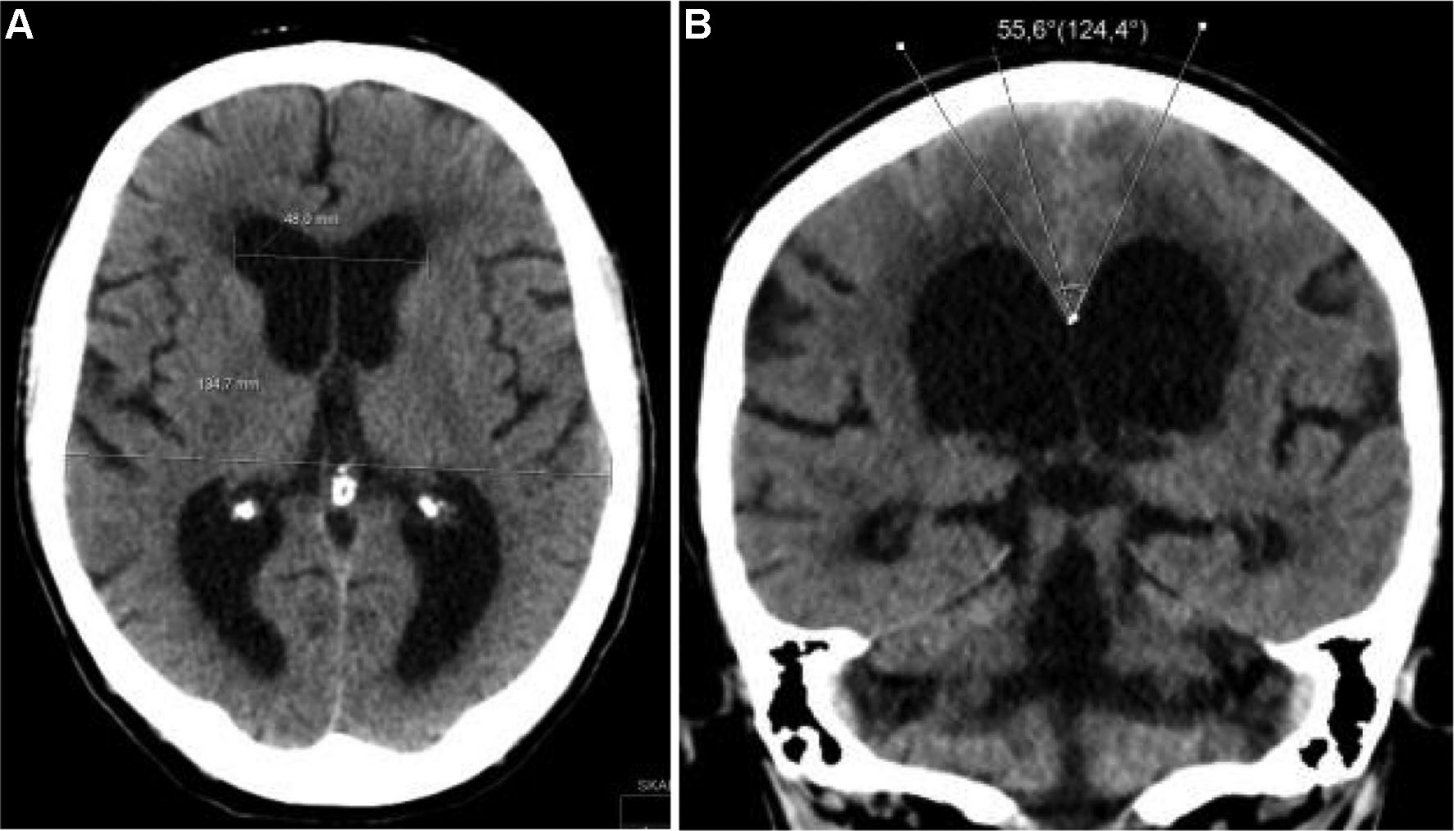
Radiological markers of NPH shown on CT scan a Evans’ index (ratio of maximum width of the frontal horns to the maximum inner skull diameter) >0.3; b callosal angle <60°, narrow medial sulci, dilated fissure Sylvii, and dilated temporal horns
